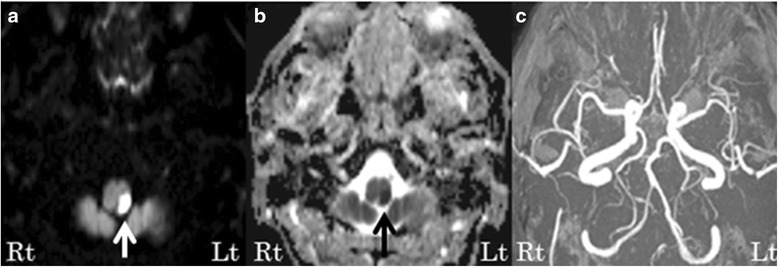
Magnetic resonance imaging (MRI) and MR angiography findings. a, b Diffusion-weighted magnetic resonance imaging (DWI) showed the hyperintensity in the left dorsolateral medulla (a, white arrow) with hypointensity on apparent diffusion coefficient (ADC)-map (b, black arrow). c MR angiography demonstrated no obvious occlusion
